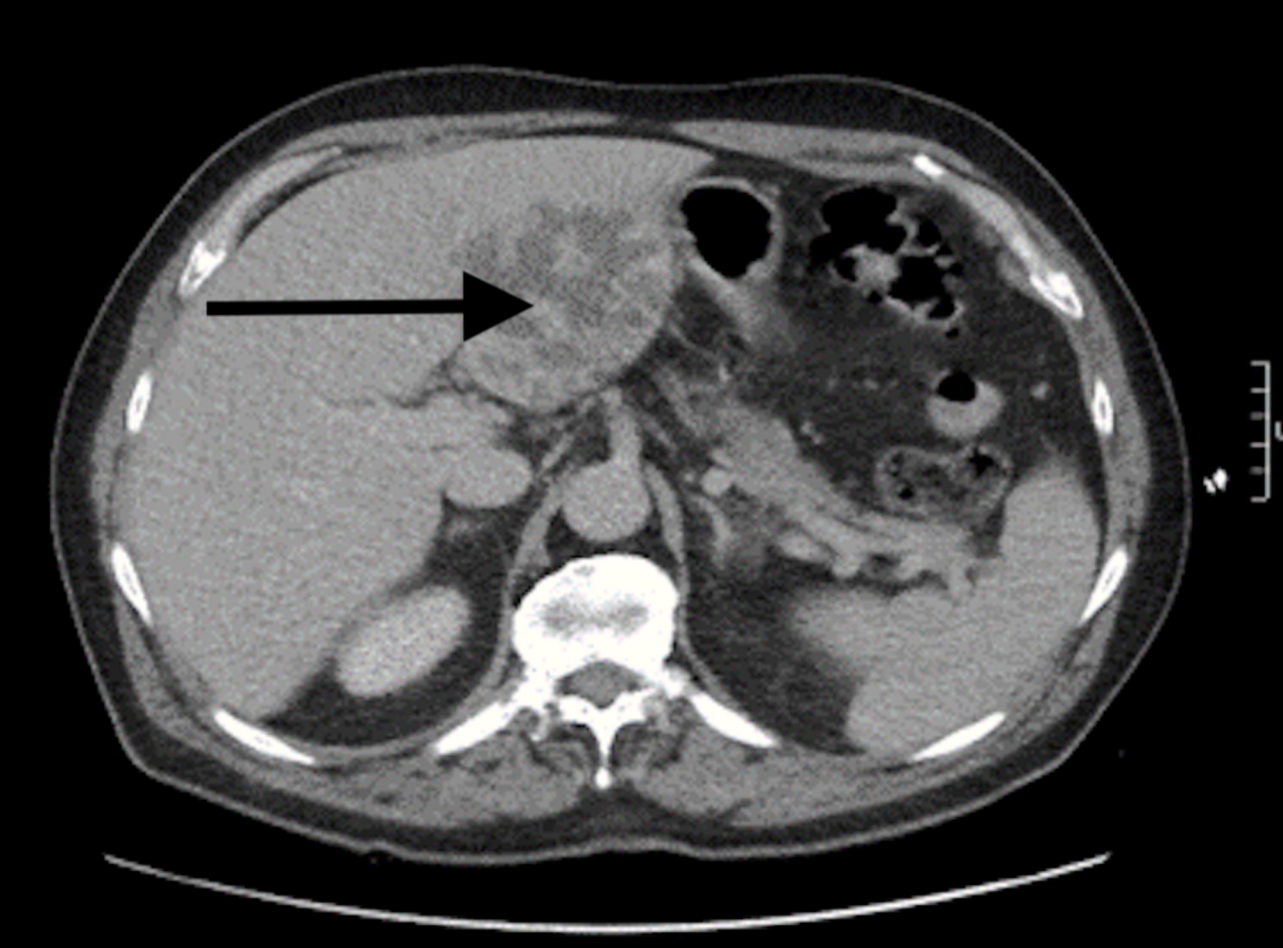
Axial computed tomography (CT) image of the abdomen showing a large, multi-loculated left hepatic abscess measuring 7.8 cm (as indicated by the arrow)
